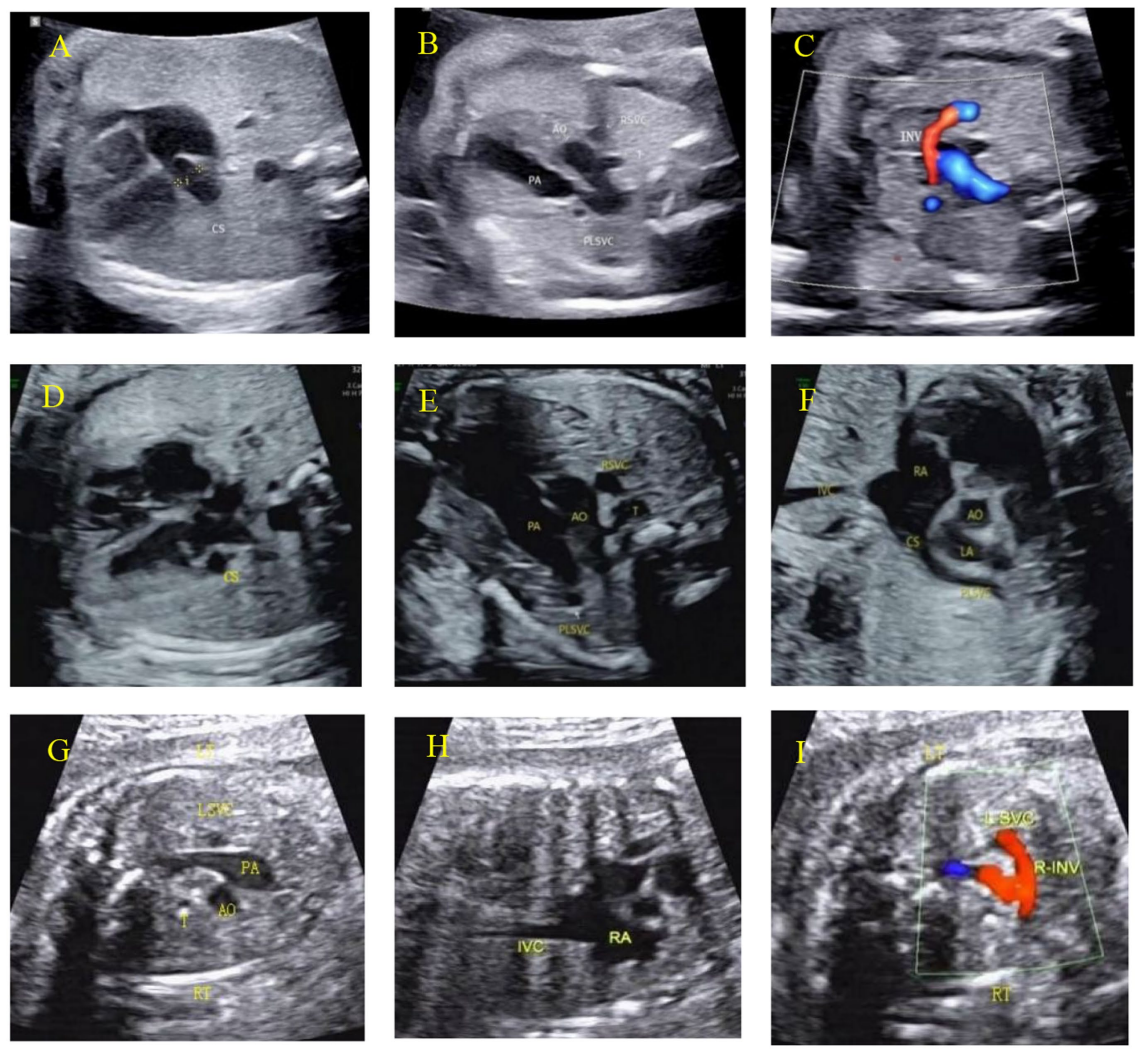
Characteristic ultrasound images of Type I PLSVC: (A–F) and (G–I). Type Ia: (A) The coronary sinus section shows the coronary sinus is widened; (B) the 3VT section shows the “four-vessel sign,” that is, the left superior vena cava appears on the left side of the pulmonary artery; (C) shows the innominate vein flowing into the superior vena cava. Type Ib: (D) the 4CV section shows the dilated coronary sinus; (E) the 3VT section shows the left superior vena cava on the left side of the pulmonary artery; (F) the paravertebral sagittal section of the neck and chest shows the left superior vena cava connecting to the dilated coronary sinus and draining into the right atrium. Type Ic: (G) the 3VT section shows the left superior vena cava on the left side of the pulmonary artery, and the right superior vena cava is not shown; (H) the long-axis section of the superior and inferior vena cava does not show the right superior vena cava; (I) CDFI shows the right innominate vein draining into the left superior vena cava on the innominate vein section.
